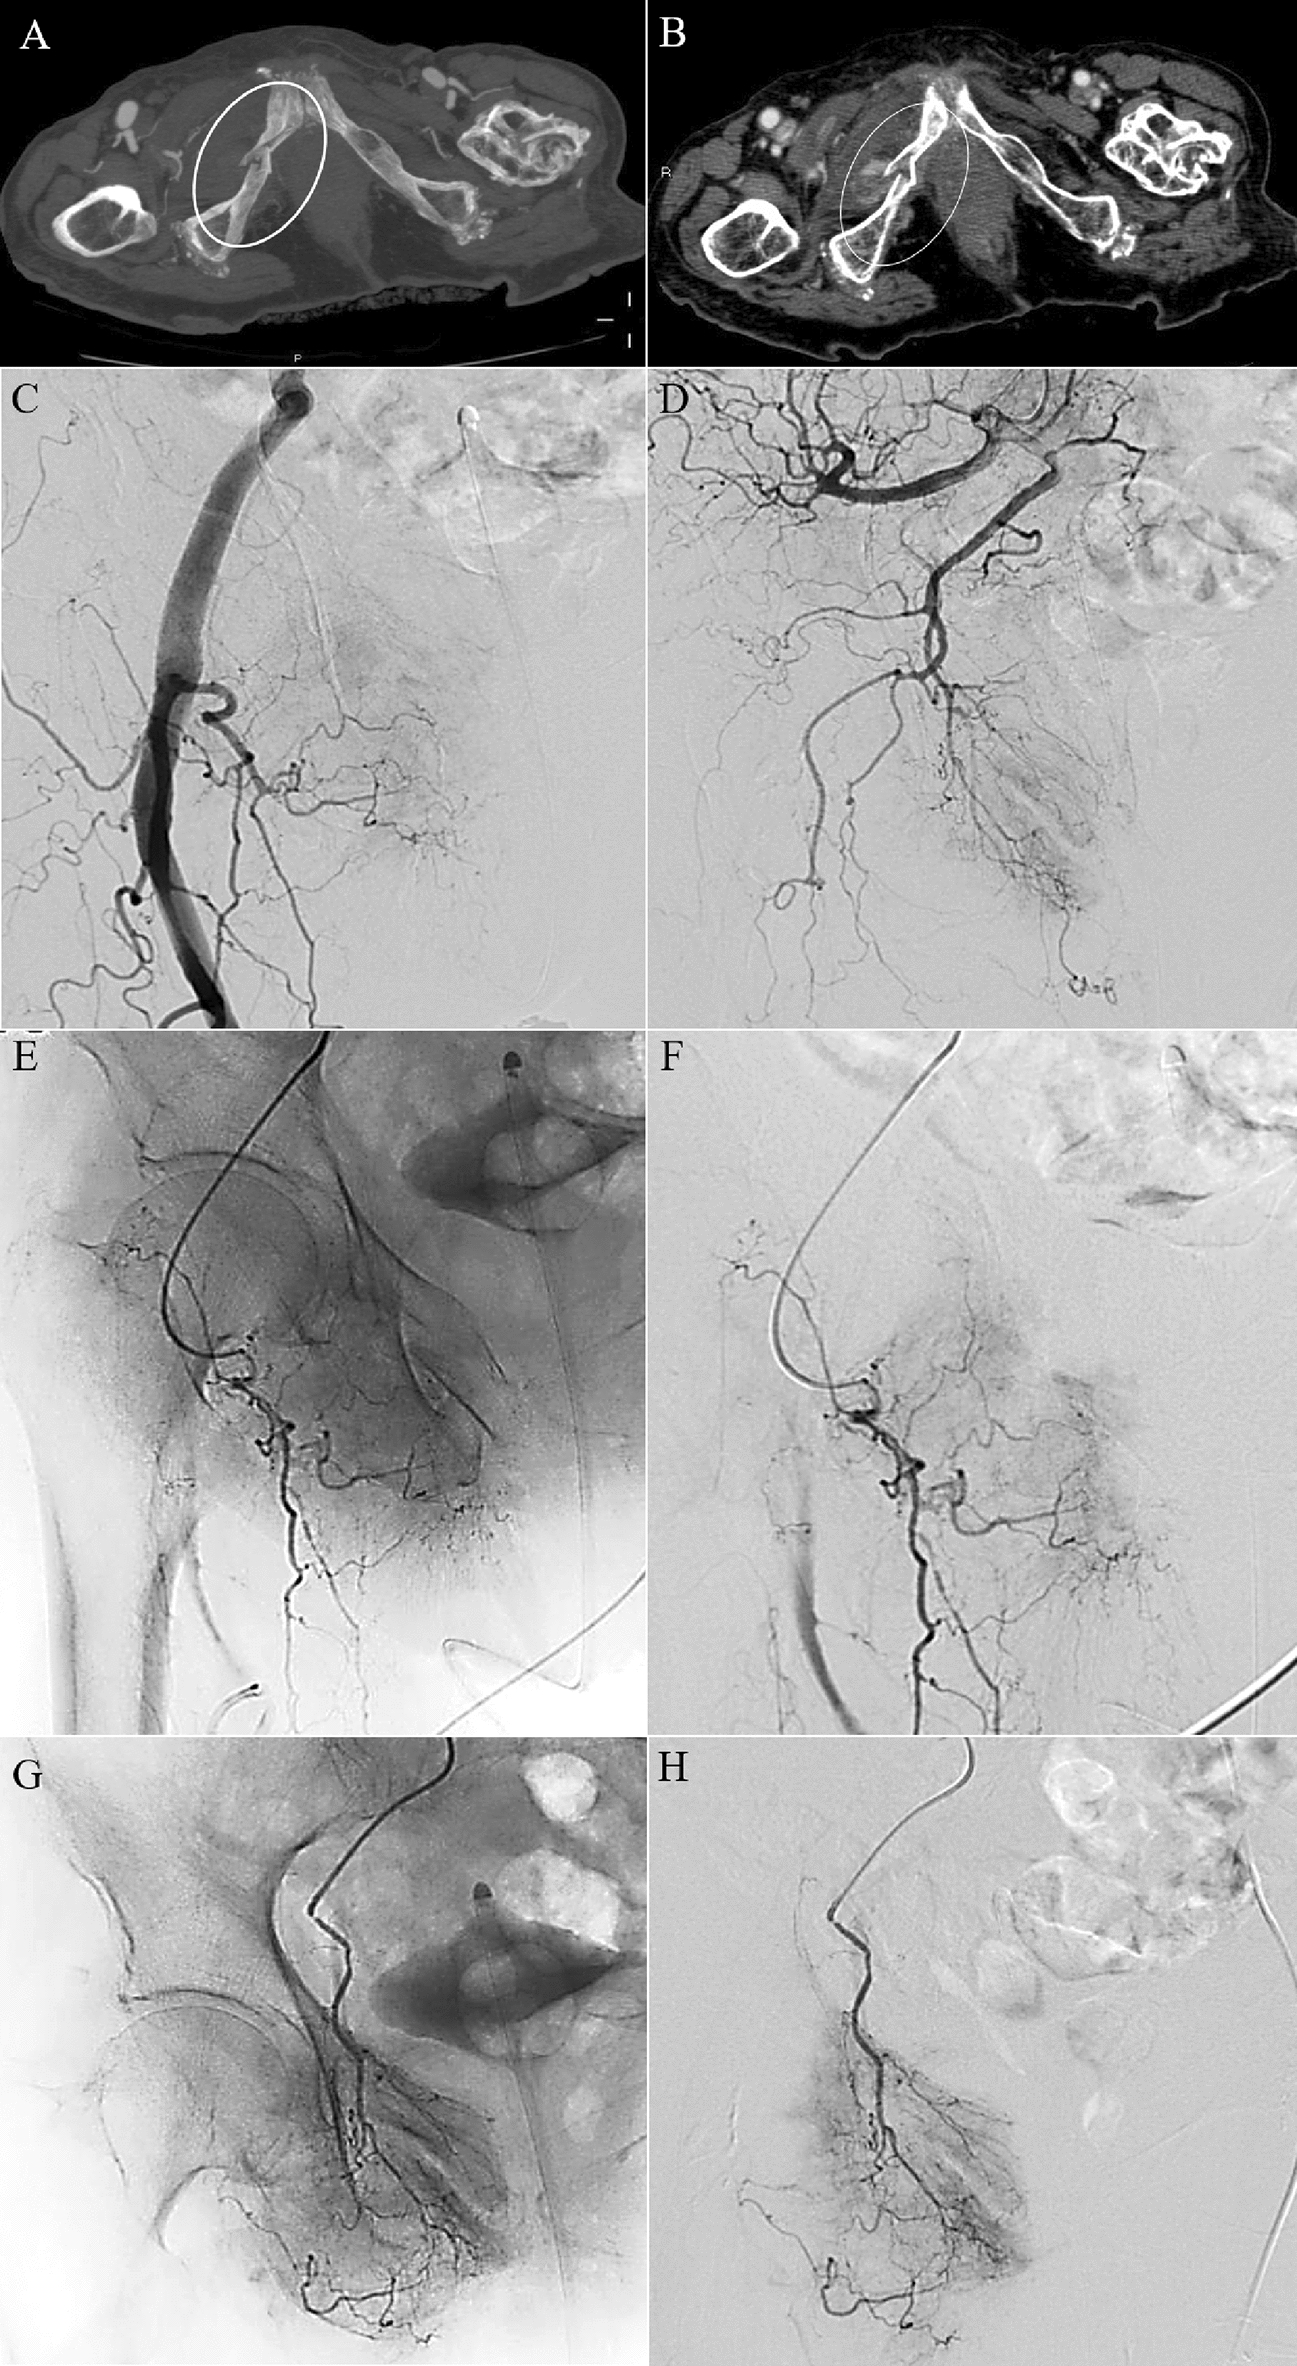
Axial CT of a stable patient with a fracture of the right pubic ramus (circled in A) presenting with a small focal region of contrast extravasation (circled in B). Proximal angiograms, from the right external iliac artery (C) and from right internal iliac artery (D), showing no signs of contrast extravasation in correspondence of the site of the findings on CT. The selective catheterizations of the suspected injured arteries based on CT findings, for the digital studies, i.e., of the right external obturator artery [non-subtracted (E) and subtracted (F) angiograms] and of the right internal obturator artery [non-subtracted (G) and subtracted (H) angiograms], showing no signs of bleeding. According to the proposed algorithm, the angiographic study was considered completed and the empiric embolization was not performed
